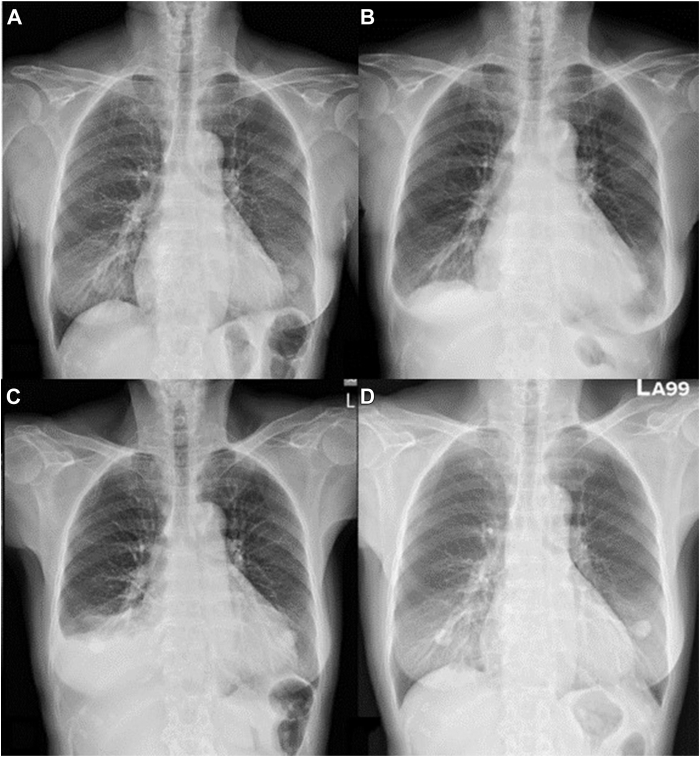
A: Posteroanterior chest radiograph before the procedure. B: Four hours after the procedure, there was no evidence of unilateral diaphragmatic elevation. C: Two weeks after cryoablation showing elevated right diaphragm. D: Two months after cryoablation showing recovery of the elevated right diaphragm.
